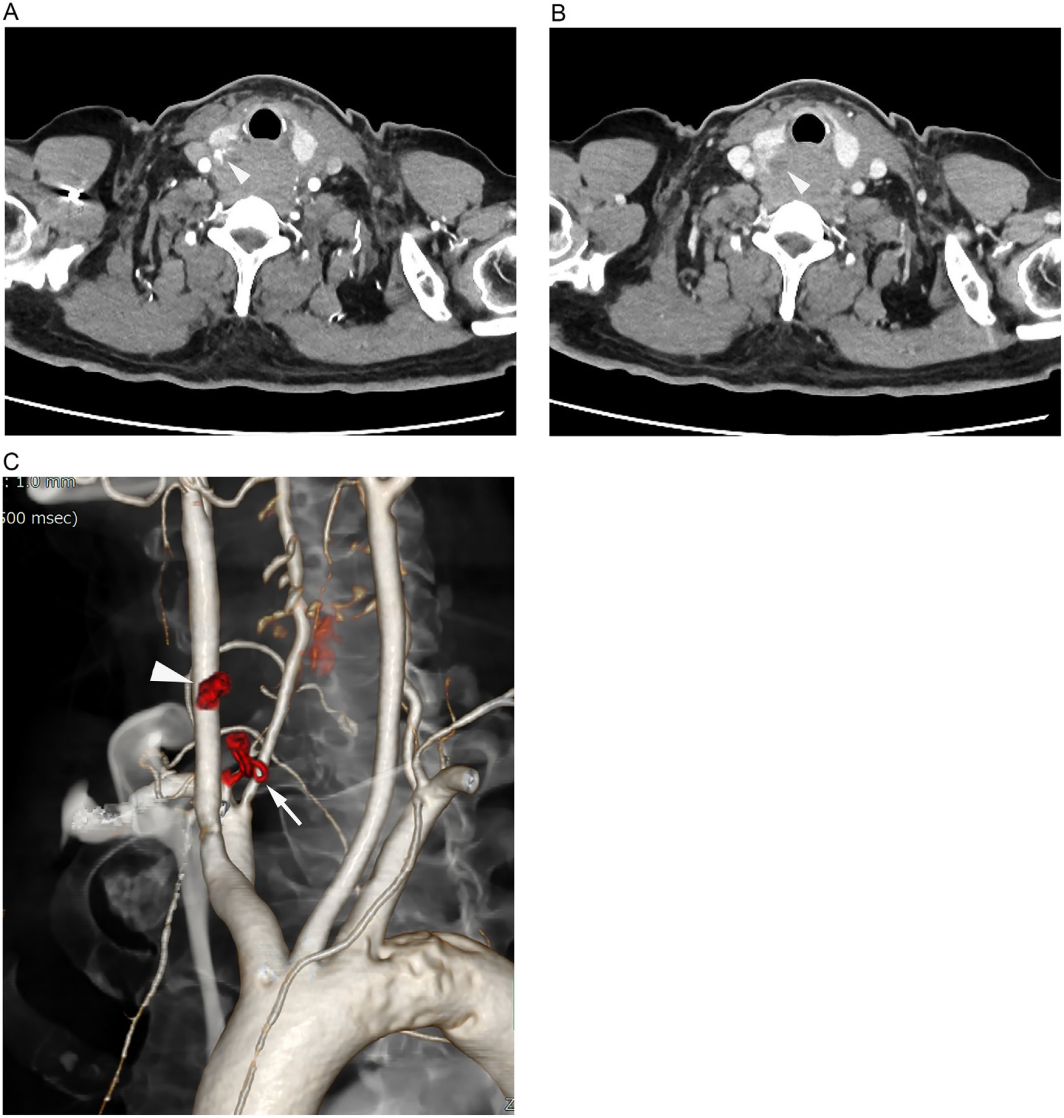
(A, B) Dynamic contrast-enhanced CT scans show extravasation of contrast media (arrowhead) posterior to the right lobe of the thyroid gland (white arrowhead), showing expansion. (C) A volume-rendering image shows the right inferior thyroid artery (white arrow) and the adjacent extravasation (white arrowhead) highlighting their proximity.
